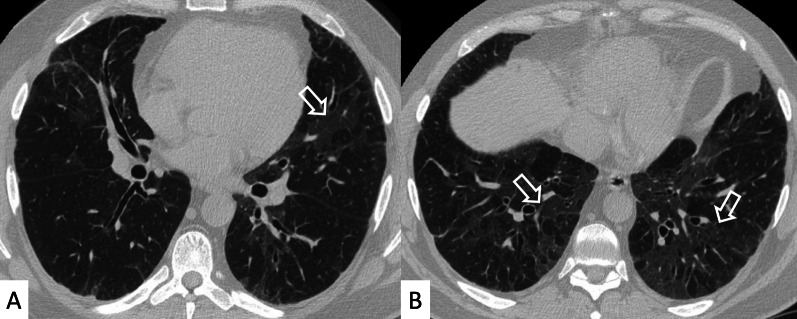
Axial HRCT images of an NSIP pattern in a patient with rheumatoid arthritis. A, B Ground-glass opacities (empty white arrows) can be appreciated through the pulmonary parenchyma. The heterogeneous distribution of ground glass regions reflects areas of inflammation—alternated to normal lung regions
